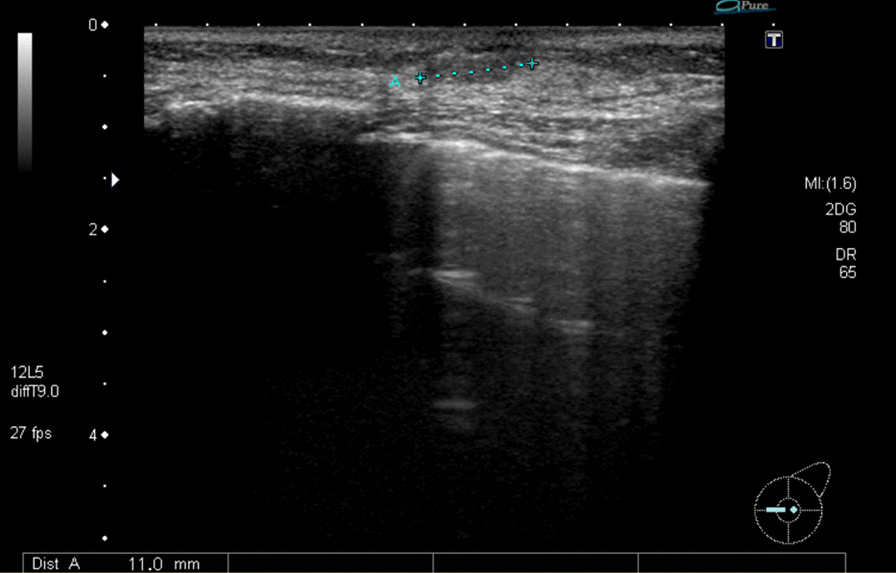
Ultrasonography (US) of the left breast. Six months after withdrawal of methotrexate, the mass had markedly shrunk like a scar
